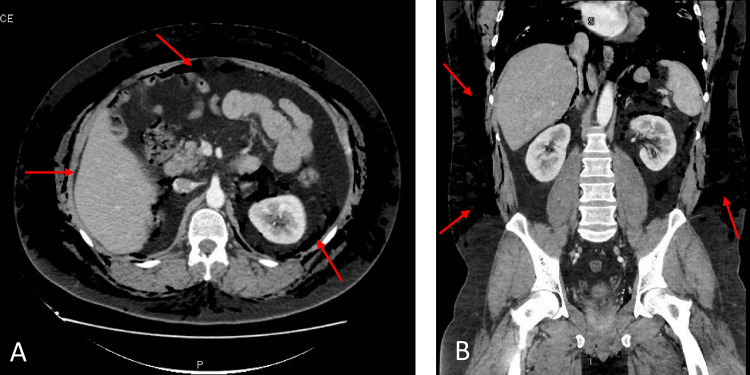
Axial (A) and coronal (B) views of CT abdomen demonstrating pneumoperitoneum and diffuse subcutaneous emphysema. The red arrows indicate the presence of free air.
